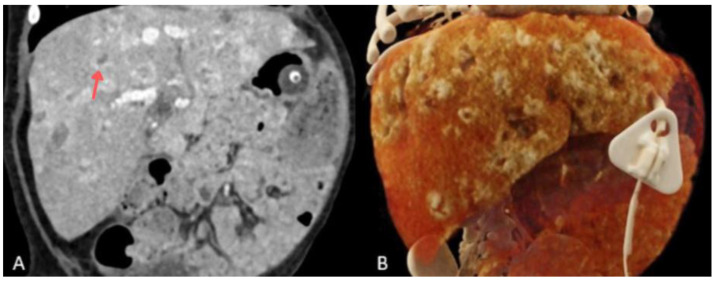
CT of the abdominal cavity with intravenous contrast in the venous phase, coronal section (A), and CT with Volume Rendering Technique (VRT) (B), performed 6 months after the initial examination (10 days prior to death). One of the lesions marked with an arrow.
